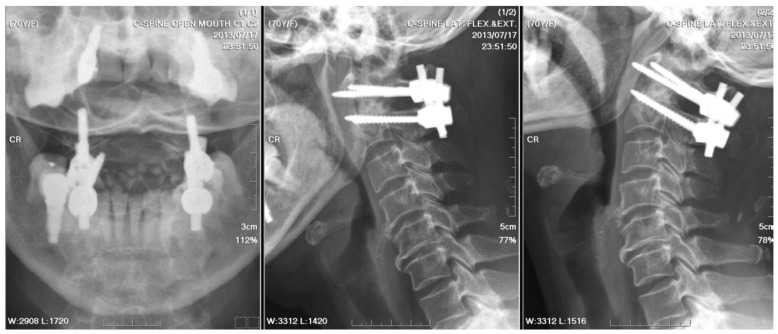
Postoperative radiographs of the cervical spine: They were checked three weeks after a five-week course of antibiotics and posterior instrumented fusion with bilateral C1 lateral mass and C2 transpedicular screw fixation. The stabilization was fair.
